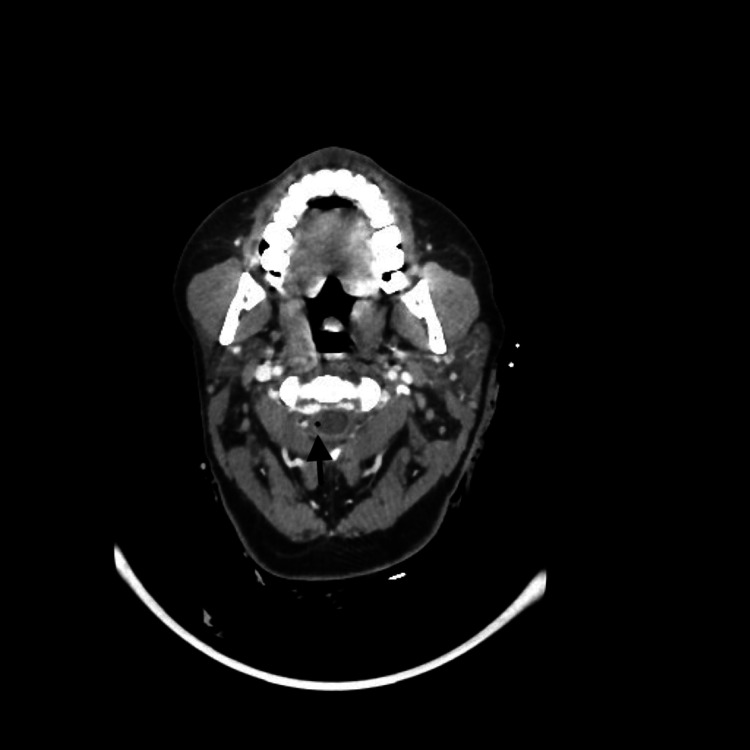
CT image showing intrathecal air focus at the C2-C3 level on the right side
